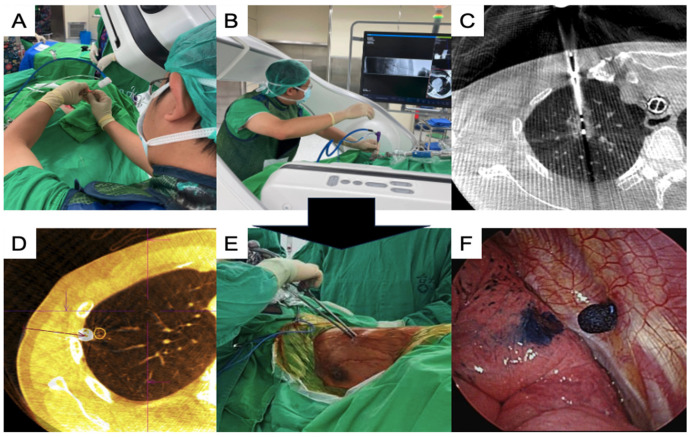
Single-stage synchronized procedures of ablation and VATS in an HOR. (A) The C-arm projects the laser cross to insert the coaxial needle for the ablation procedure; (B) insertion of the ablation needle using arm support under a progressive view augmented fluoroscopy; (C) post-ablation CT showing the ablation zone while the needle was still inside the lesion; (D) confirmation CT scan for checking the stamped area alignment with the actual lesion location; (E) thoracoscopic surgery using a uniportal approach; (F) the dye-stamped area was identified to guide thoracoscopic resection. CT, computed tomography; VATS, video-assisted thoracic surgery; HOR, hybrid operating room.
